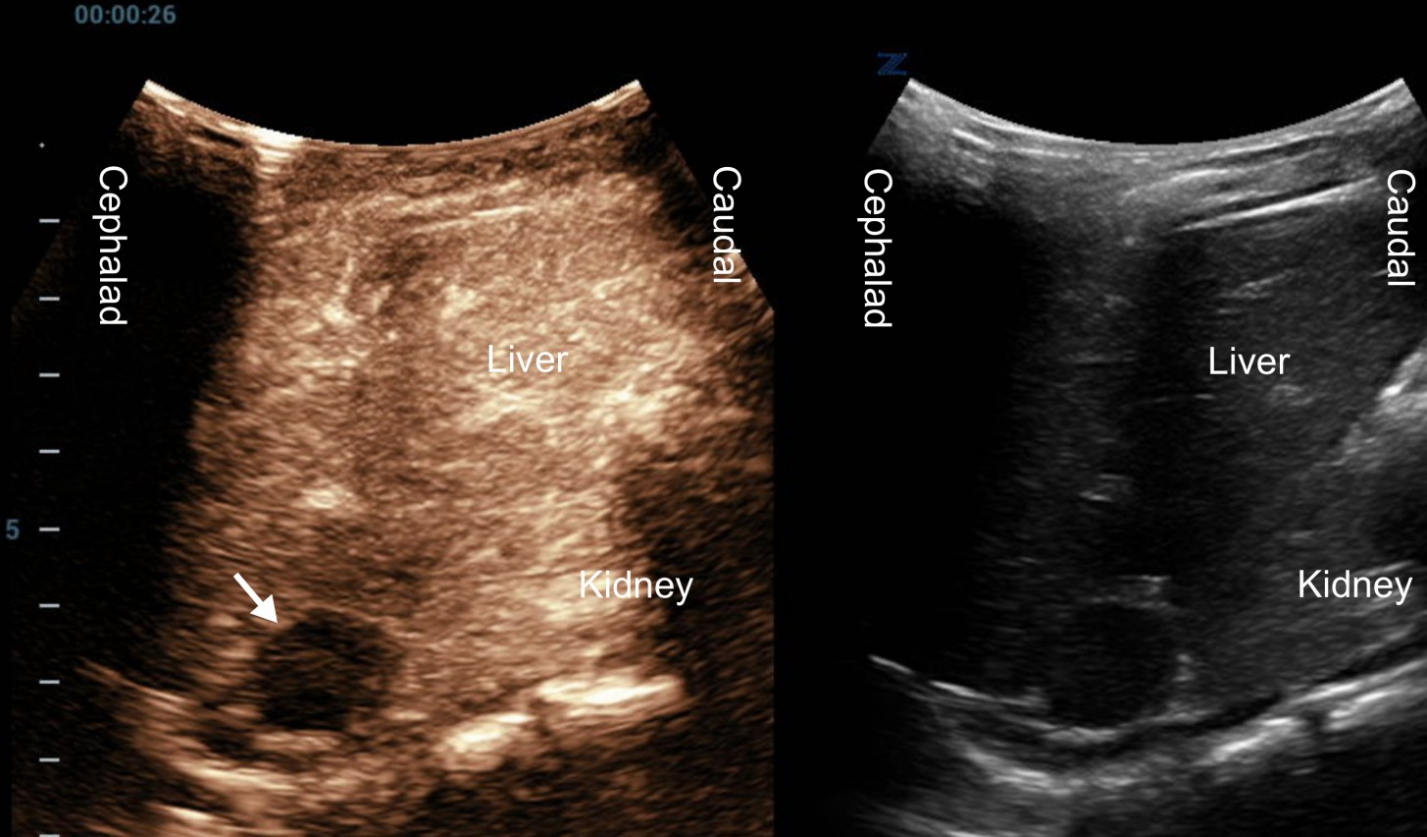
A dual-mode contrast-enhanced ultrasound (CEUS) image (left side - CEUS; right side - grayscale), at the arterial phase, of the oval finding (arrow) at the adrenal cephalad area. The kidney appears in a longitudinal view. This finding shows no marked enhancement, suggesting a blood clot.
